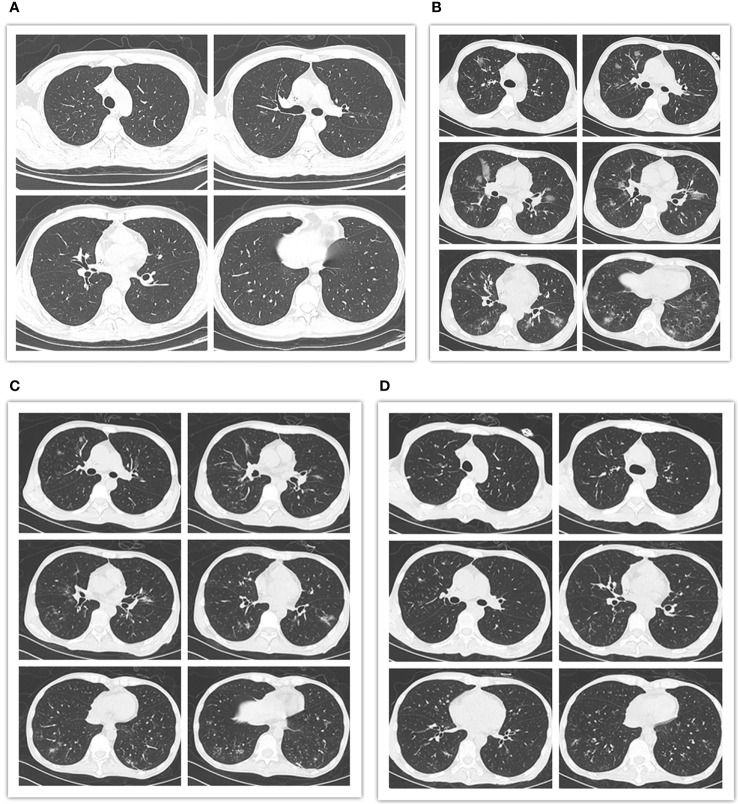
Pre- and post-treatment chest computed tomography (CT) scans. (A) CT images obtained 3 days prior to tislelizumab and azacitidine therapy showing no signs of infection. (B) CT images obtained 3 days after initiation of the combination therapy revealing bilateral new ground glass opacities. (C) CT images obtained 5 days after the administration of methylprednisolone showing obvious improvement in the bilateral lobe lesions. (D) CT images obtained 1 month following initiation of methylprednisolone therapy. The bilateral lobe lesions were completely absorbed and disappeared.
